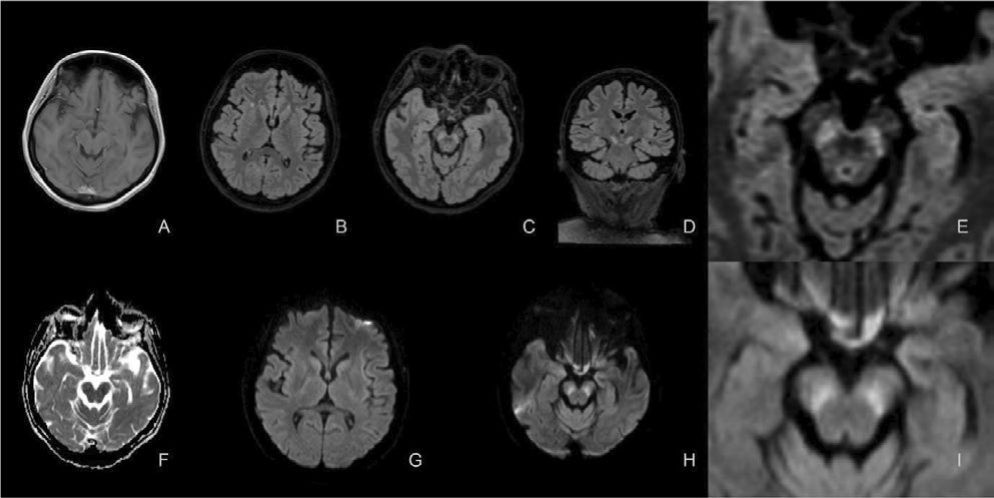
FIGURE 1 Cerebral MRI showing bilateral extensive substantia nigra lesions, with hyperintense signal in T2/FLAIR and restriction of diffusion on DWI.
